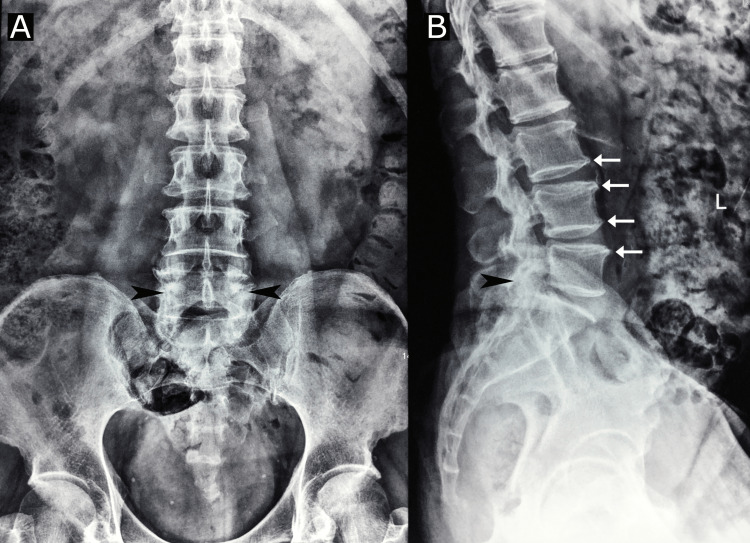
Lumbar spine radiographs Anteroposterior (A) and lateral (B) radiographs taken prior to presentation to the chiropractor reveal facet sclerosis at L5/S1 (arrowheads), while the lateral radiographs (B) reveal anterior vertebral osteophytes (arrows) and marked reduction in lumbar lordosis. These findings are consistent with mild degenerative spondylosis of the lumbar spine. There are no signs of serious pathology.
